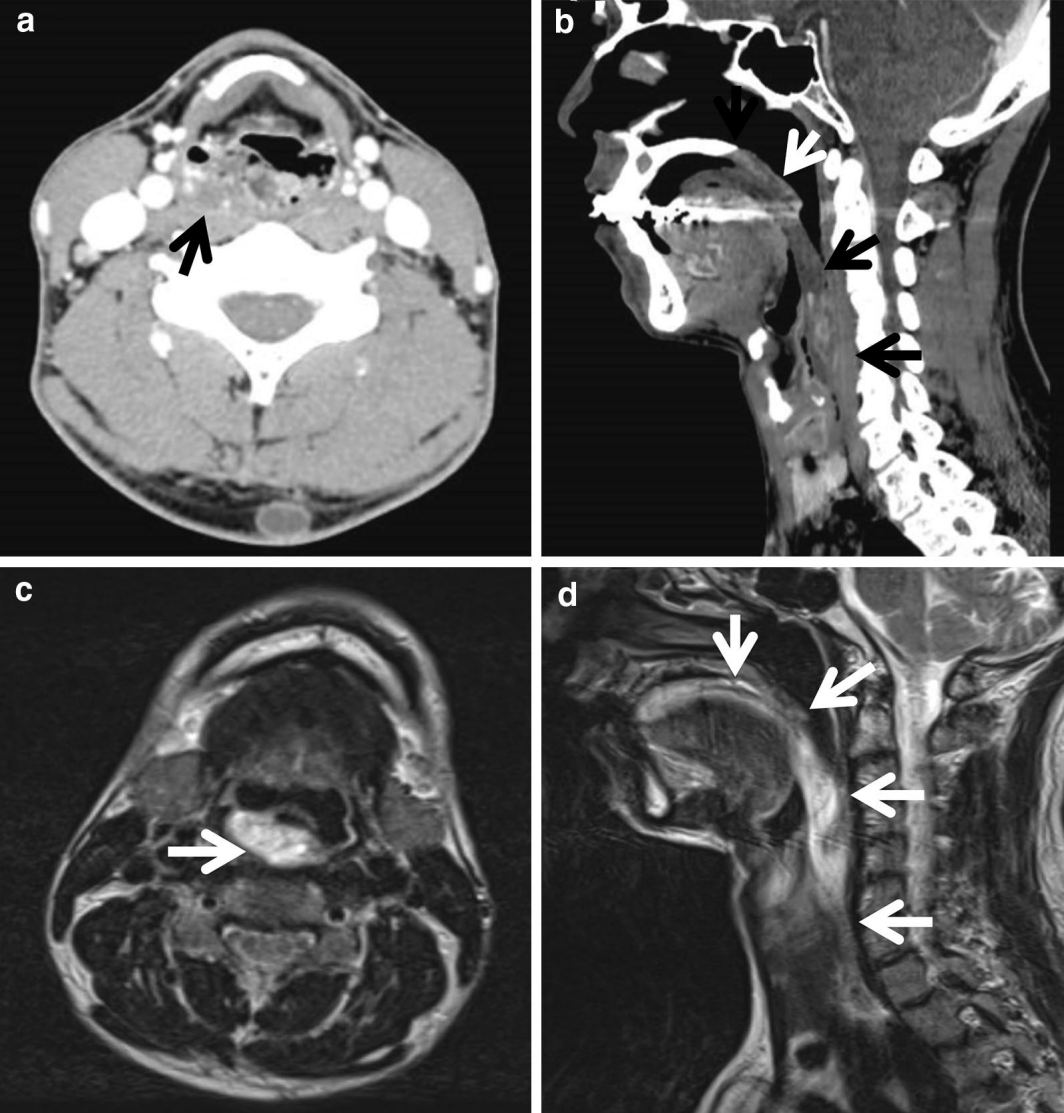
Radiological findings. a, b: Contrast-enhanced computed tomography scans revealed that the tumor (*arrow*) occupied the oral cavity to the esophagus (a axial, b sagittal). c, d: T2-weighted magnetic resonance imaging revealed a high-intensity area within the tumor (*arrow*) (c axial, d sagittal)
